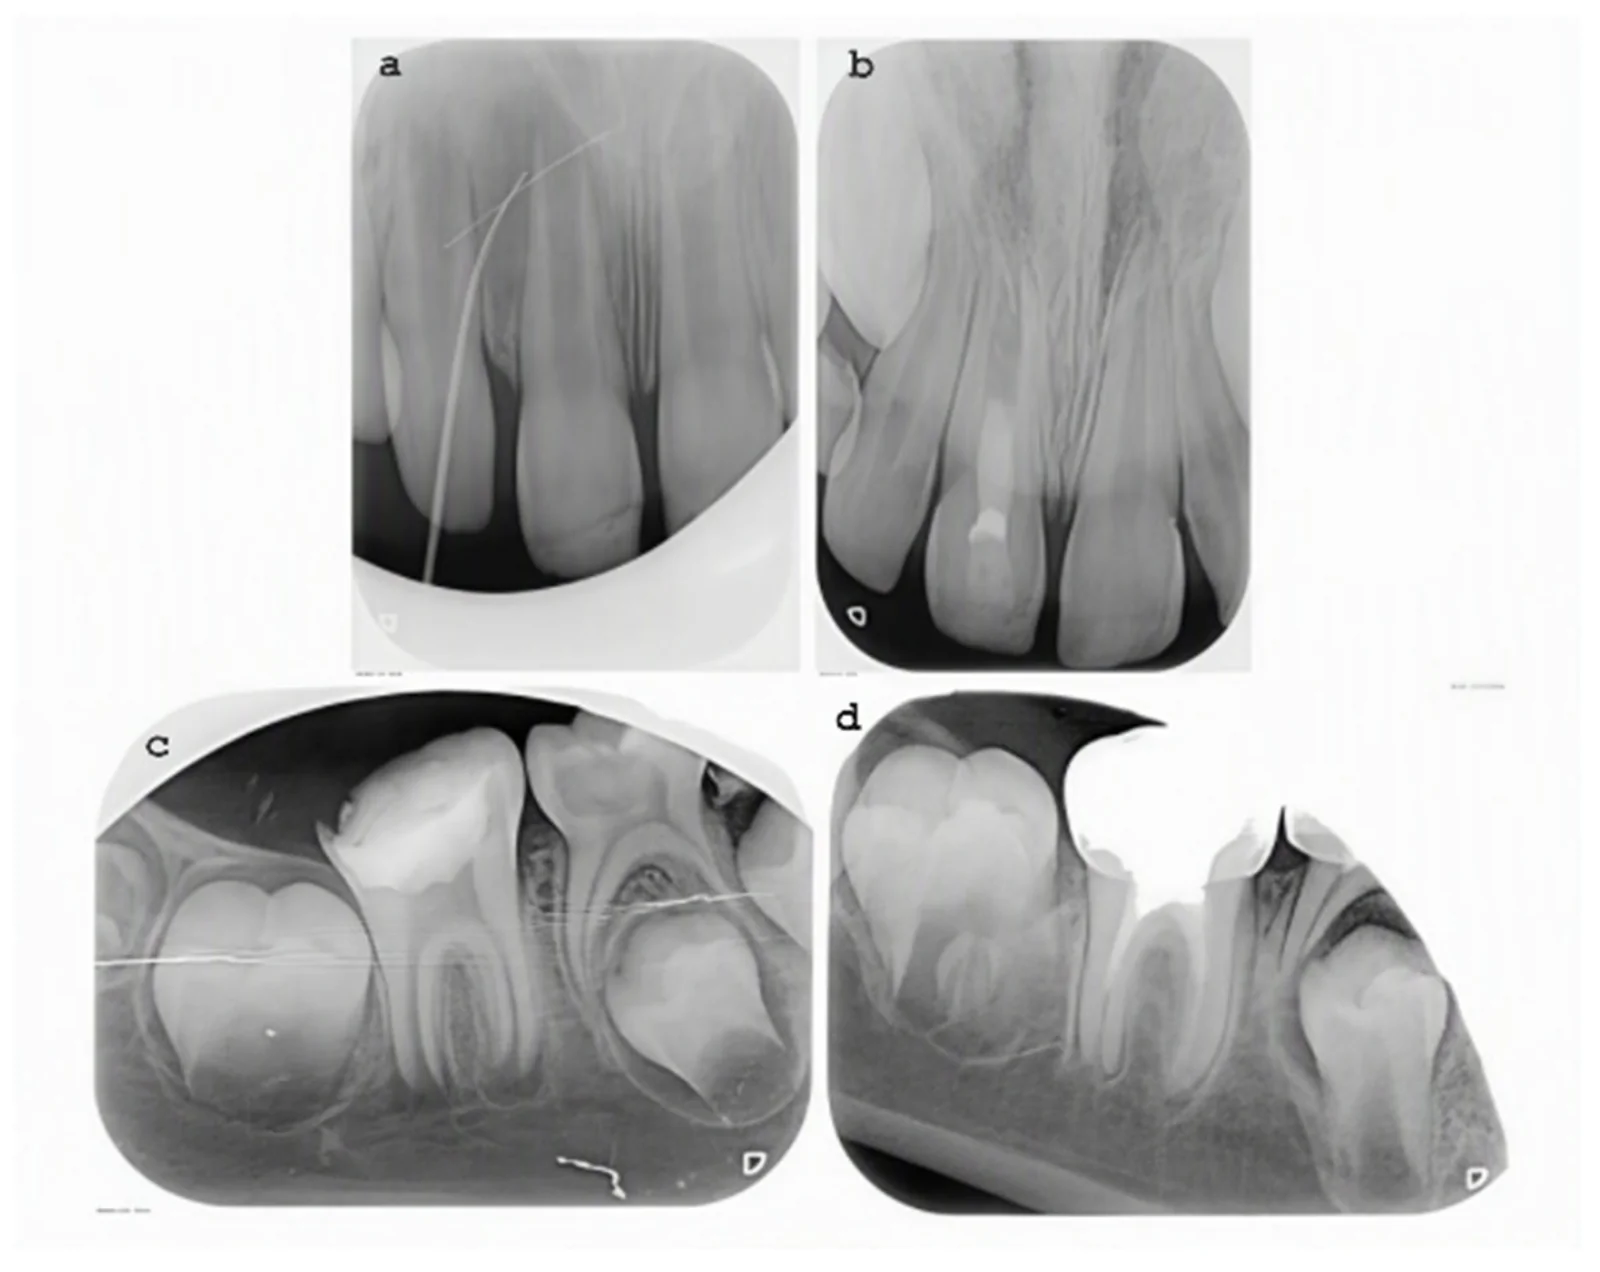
Radiographic apical healing following regenerative endodontic treatment. Necrotic maxillary incisor with traumatic etiology (a) and 12 months after treatment (b). Necrotic mandibular first molar with carious etiology (c) and 12 months after treatment (d).
